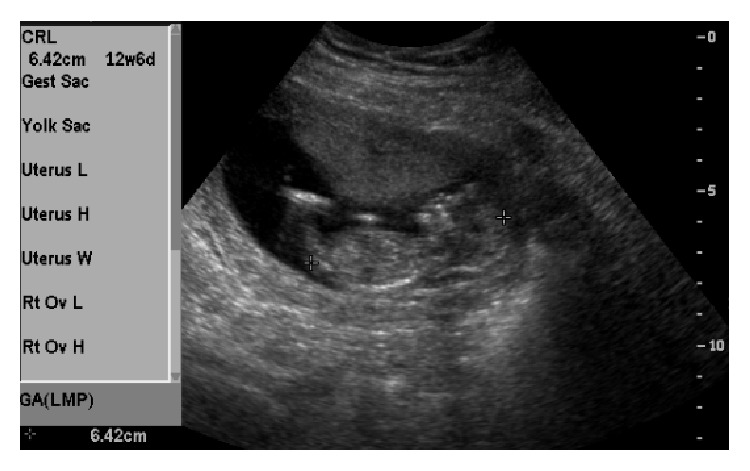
Patient number 2, pelvic sonogram on 4/2/15: showing IUP at 12 6/7 wks, while both Essure inserts are still in place.
